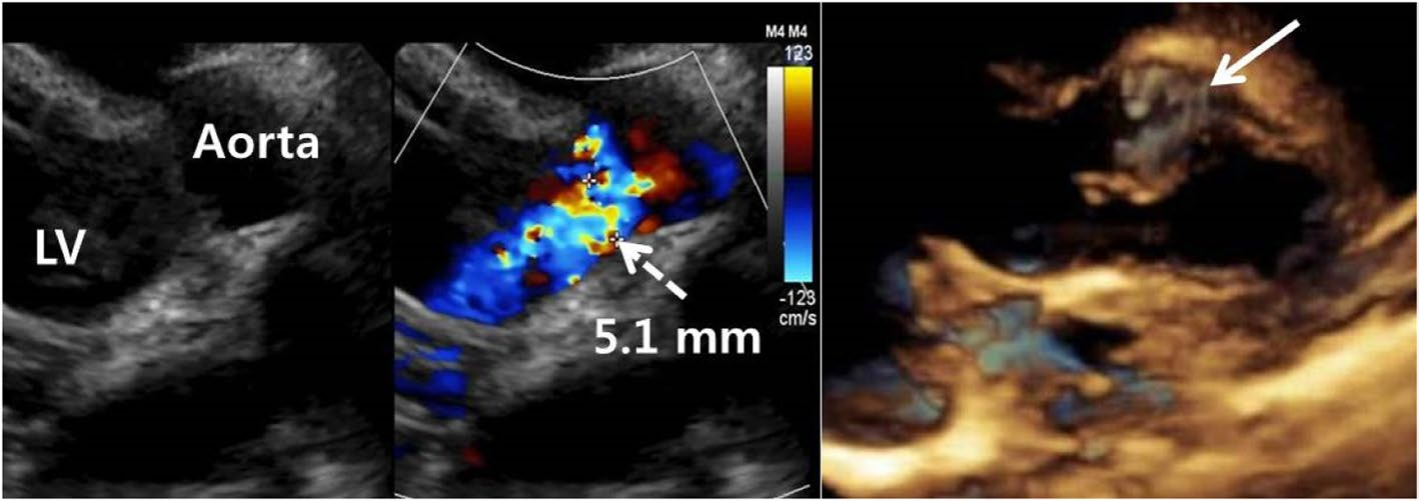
Transthoracic echocardiography. Aortico-left ventricular tunnel (bold arrow) with moderate AR about 5.1 mm color width (dotted arrow). AR: aortic regurgitation, LV: left ventricle
